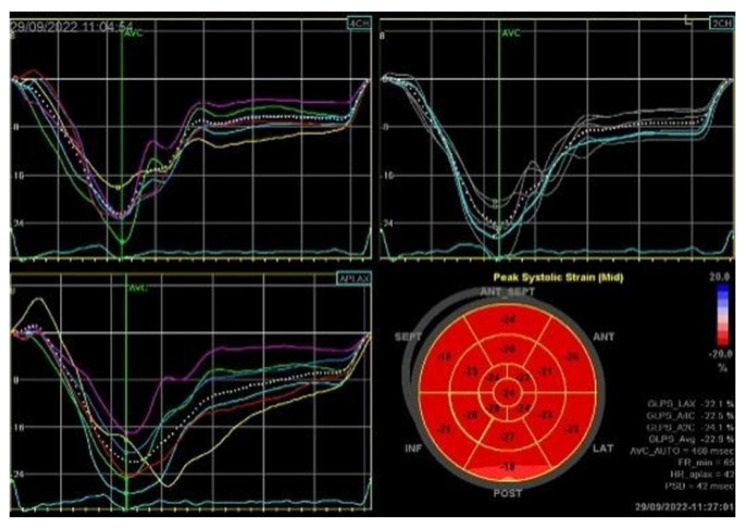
2D speckle-tracking echocardiography used as a tool for assessing left ventricular systolic performance (GLS average −22.9%), with some degree of dispersion in longitudinal strain peaks.
